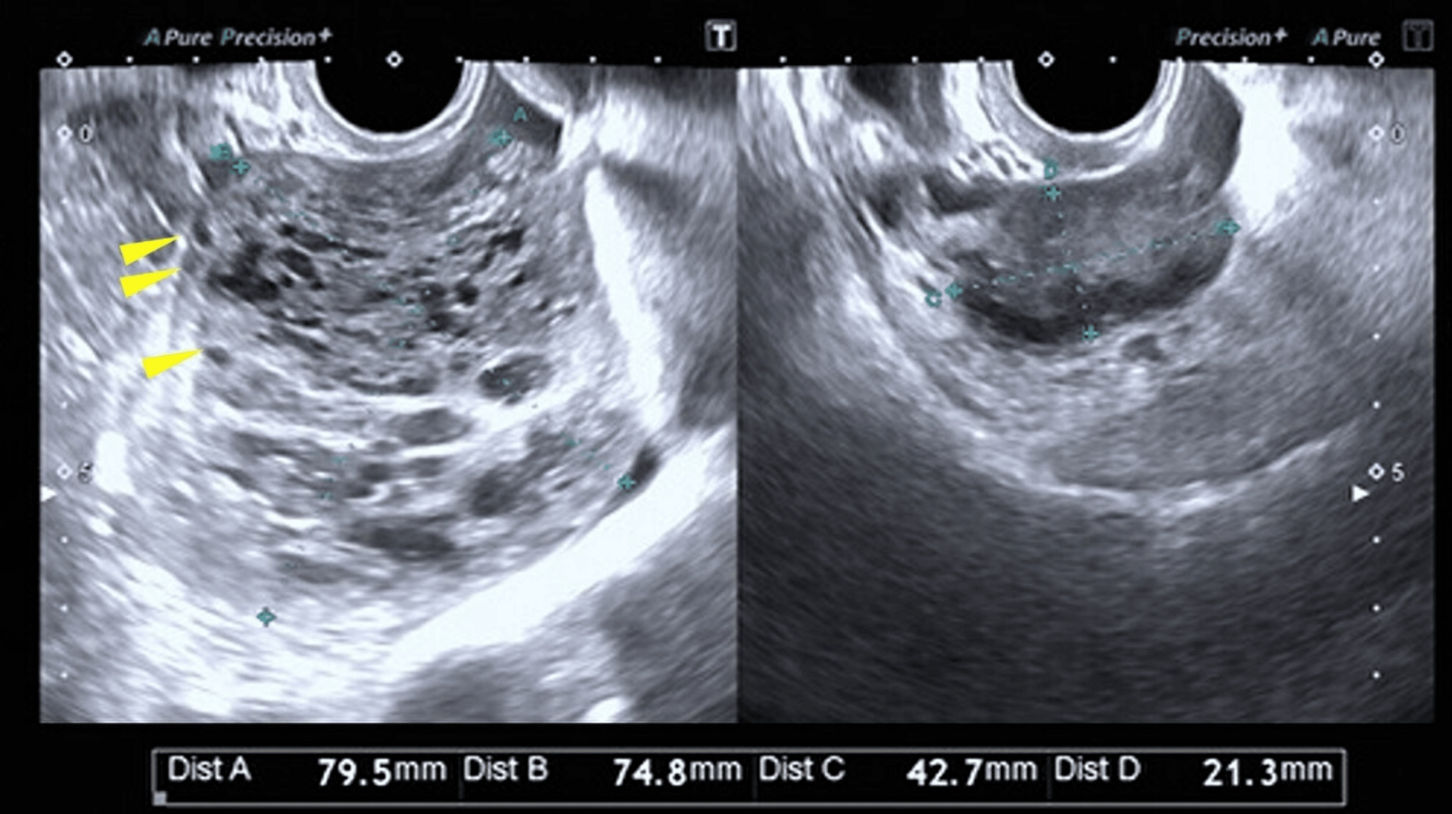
Transvaginal ultrasonography of both ovaries. The image on the left shows the right ovary, measuring 79.5 × 74.8 mm, with multiple small cystic structures along the inner margin and mottled hypoechoic areas within the parenchyma. Arrowheads indicate the small cystic structures. The image on the right shows the normal left ovary, measuring 42.7 × 21.3 mm.
